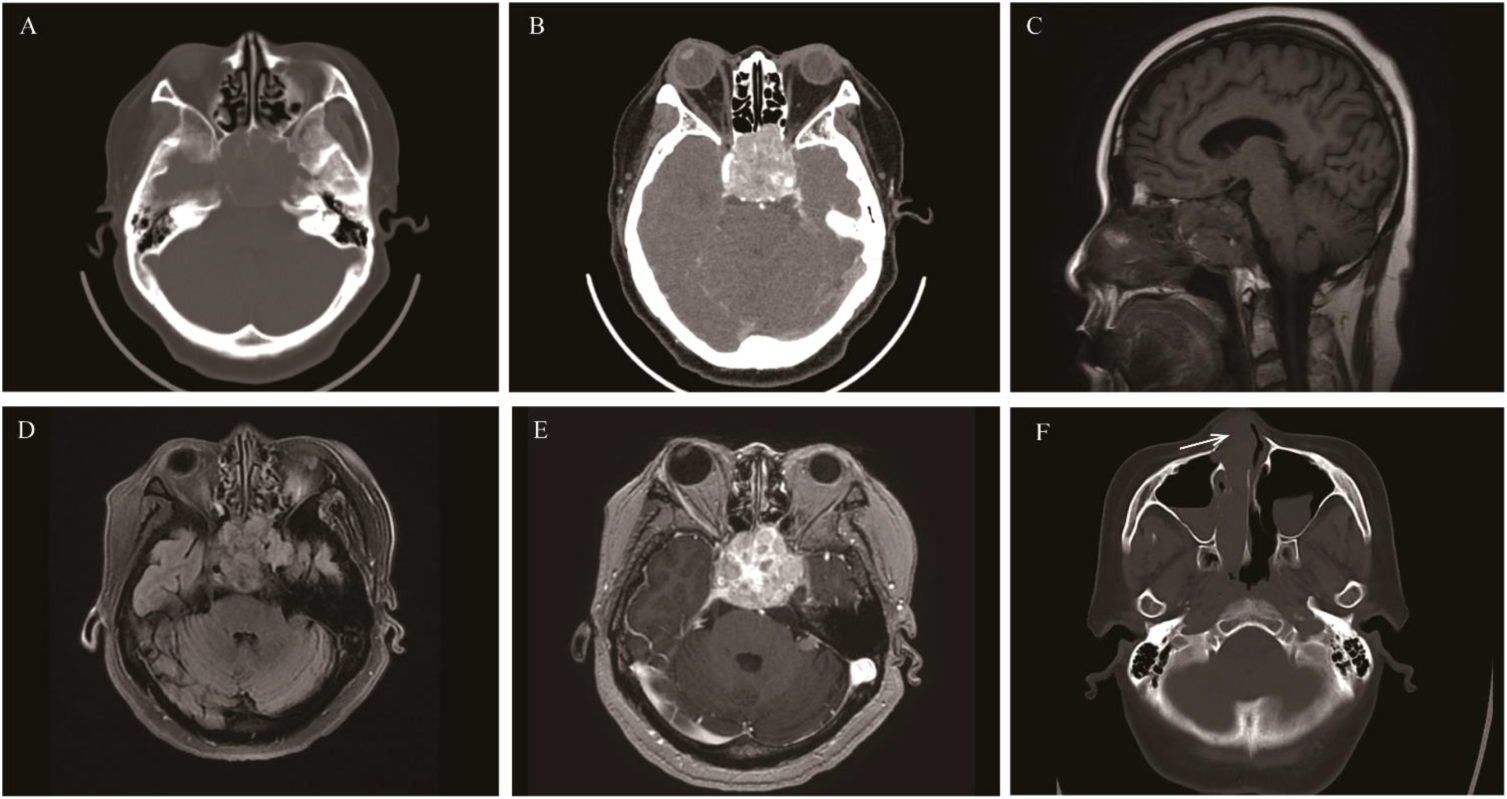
Sinonasal metastasis: Patient 1: A soft tissue mass involving the upper part of the nasal cavity, sphenoid sinus, ethmoid sinus, cavernous sinus, and clivus, with obvious bone destruction on CT (A, B). It showed heterogeneous isointensity on T1WI (C), hyperintensity on T2WI (D), and marked enhancement on contrast-enhanced MRI (E). Pathology confirmed that it was derived from clear cell renal carcinoma. Patient 2: A mass in the right nasal vestibule (white arrow), which was pathologically confirmed to be derived from hepatocellular carcinoma (F).
